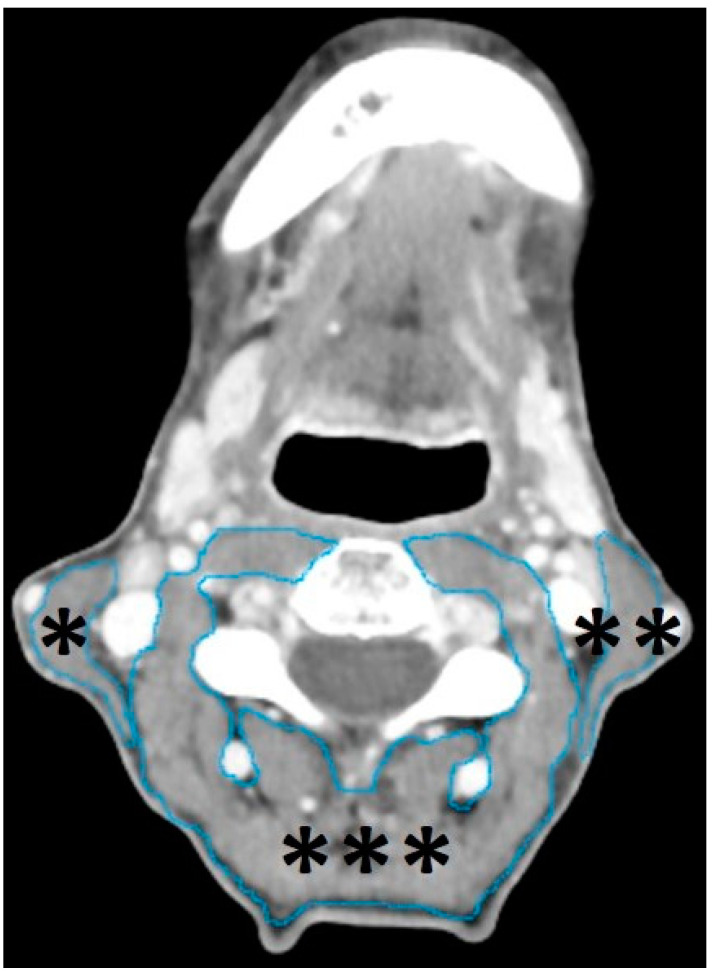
Example of segmented head and neck musculature in a staging CT of a 56-year-old male HNSCC patient with a tumor of the hypopharynx (not depicted), staged cT3 cN0 cM0. The manual slice-by-slice segmentation was performed in the axial plane using the “paint on slice” tool provided by mint Lesion™ (Mint Medical GmbH, Heidelberg, Germany, version 3.8.1) at the level of the third cervical vertebra, as previously proposed [32], for the right SCM (*), the left SCM (), and the PVM (*), which included the following muscles: trapezius, longus capitis, splenius capitis, semispinalis capitis, longissimus capitis, levator scapulae, and longus colli.
